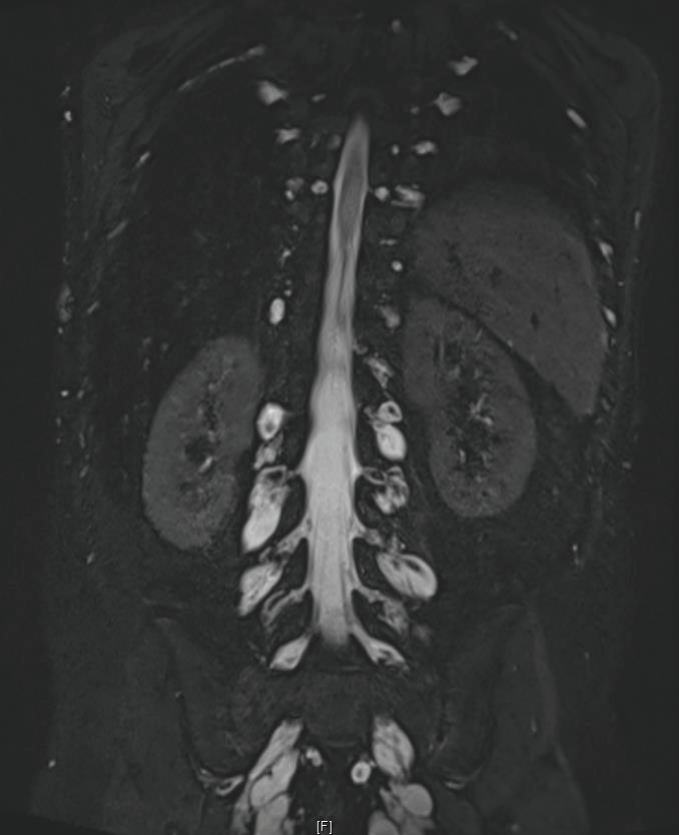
Lumbar neurofibromas: MRI sequence, coronal T2 STIR Gadolinium.
